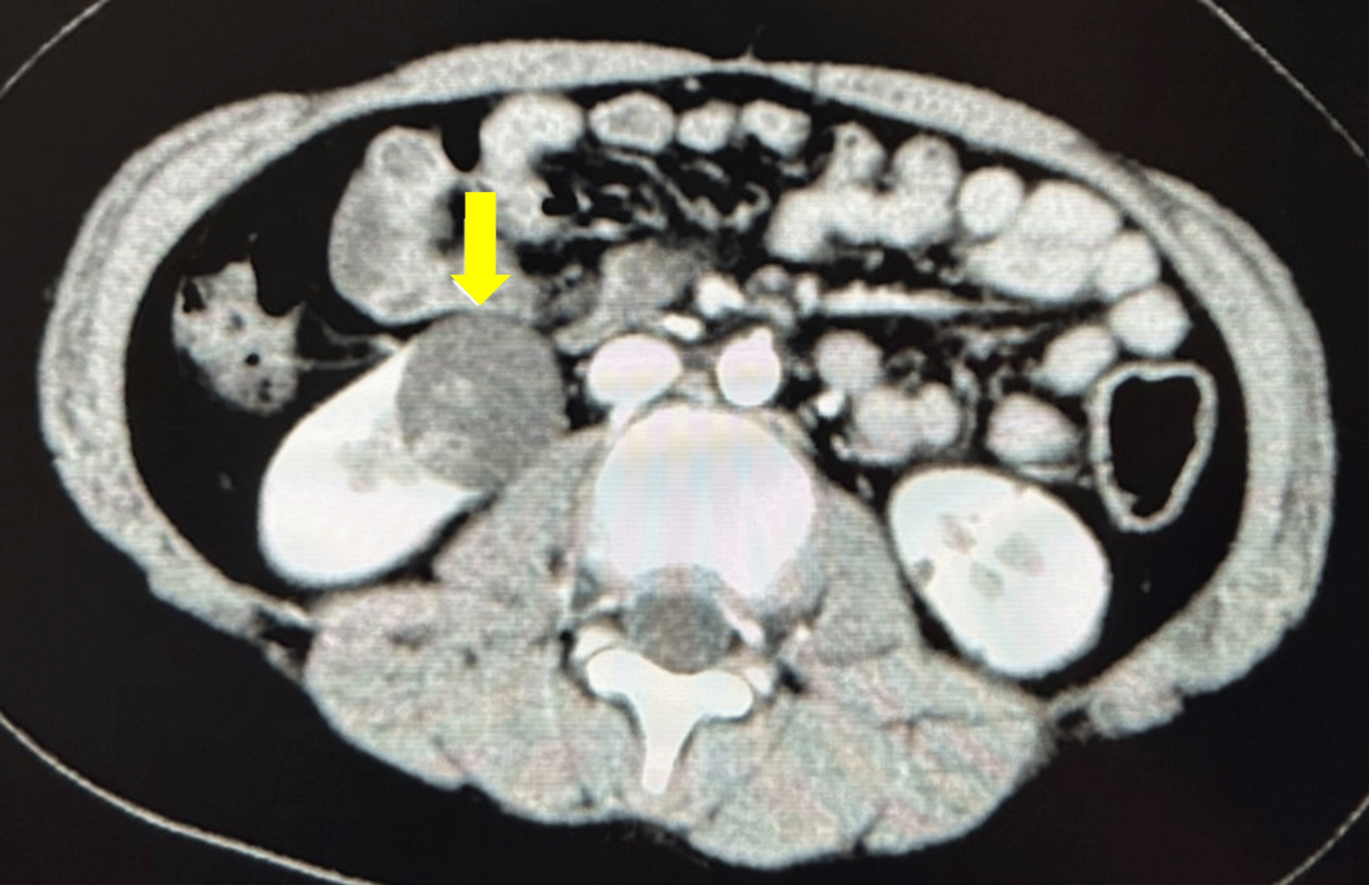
Contrast-enhanced CT Contrast-enhanced CT showing a cystic lesion (32 mm) with an enhancing solid component (16 × 12 mm) suggestive of malignancy.
